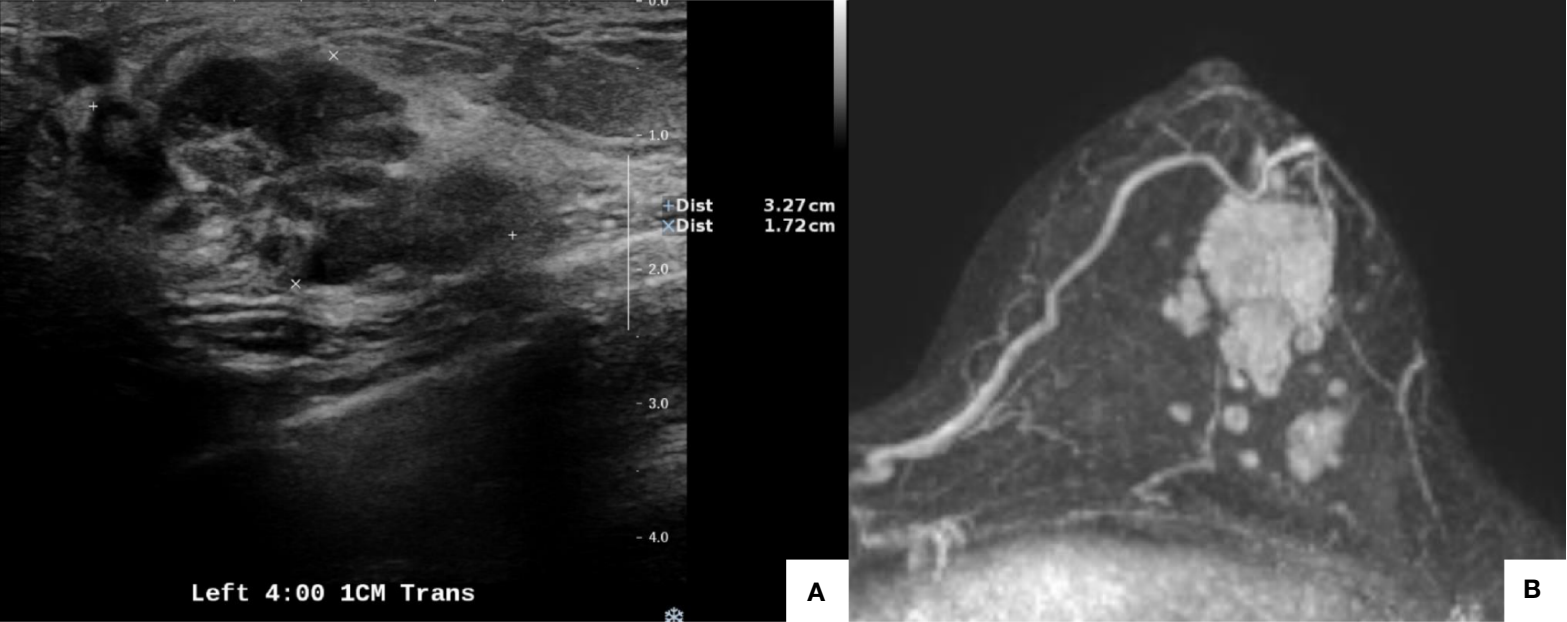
Pretreatment imaging evaluation. Ultrasonography showed an irregular hypoechoic mass at the 4-o’clock position, 1 cm from the nipple, measuring 3.9 × 3.3 cm. Malignancy was confirmed with a biopsy (A). Magnetic resonance imaging revealed a mass (3.4 × 2.2 cm) at the 4-o’clock position, 1 cm from the nipple in the left breast, which was concordant with the biopsy-confirmed malignancy. Multiple enhanced nodules (1.4 cm in the longest diameter) were observed, and the total extent measured 5.8 cm (B).
